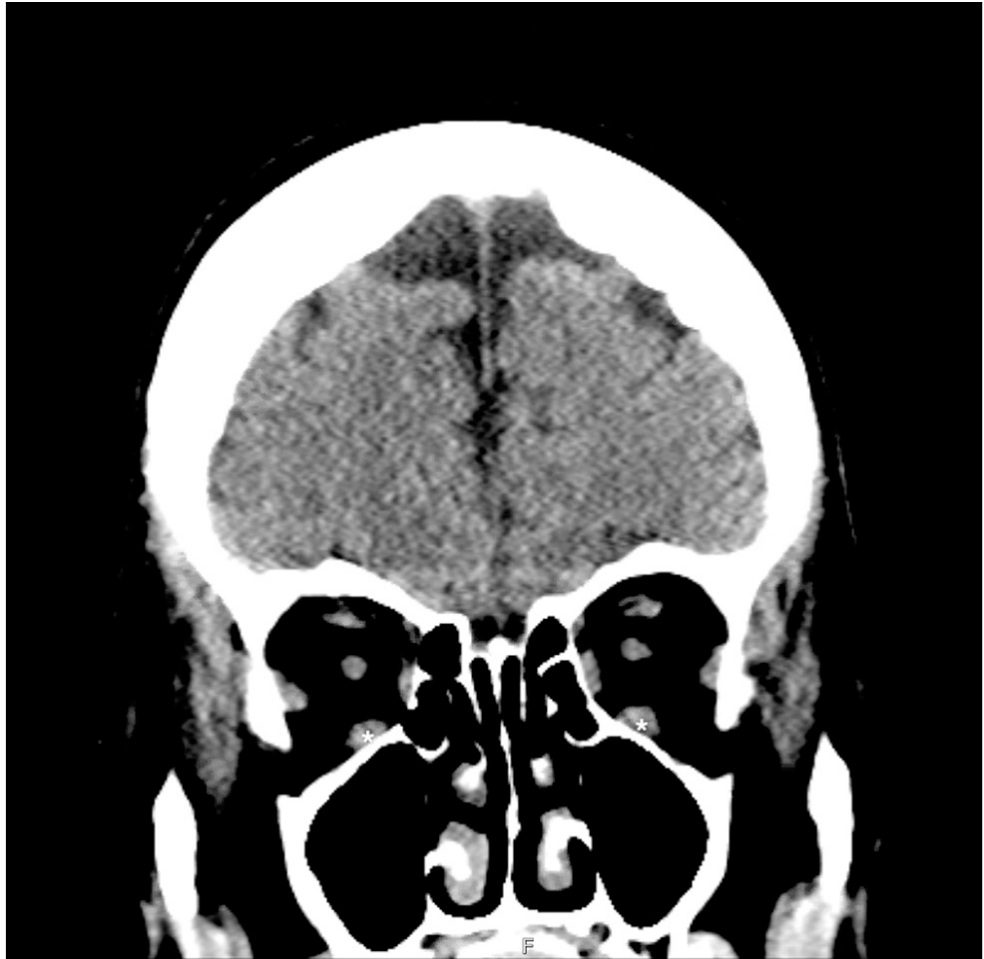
Selected CT coronal image of the head without contrast demonstrating mild enlargement of the inferior recti muscles (asterisks) CT: computed tomography
